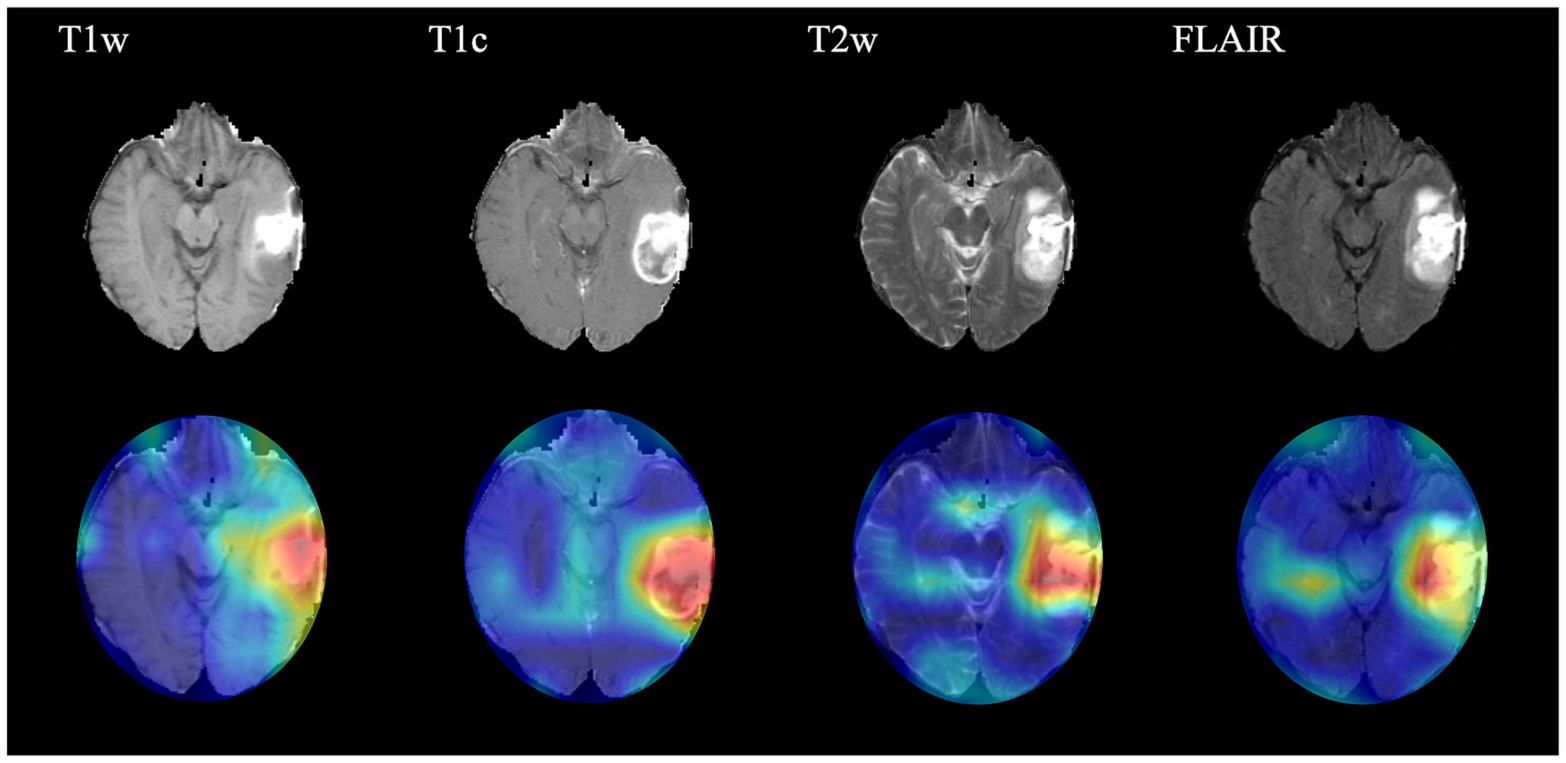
Class activation maps (CAMs) corresponding to four magnetic resonance imaging sequences of a random patient. The CAMs highlighting regions that contribute more to the prediction of glioma type. T1w, T1-weighted; T1c, T1-weighted gadolinium contrast-enhanced; T2w, t2-weighted; FLAIR, T2-weighted fluid-attenuated inversion recovery.
